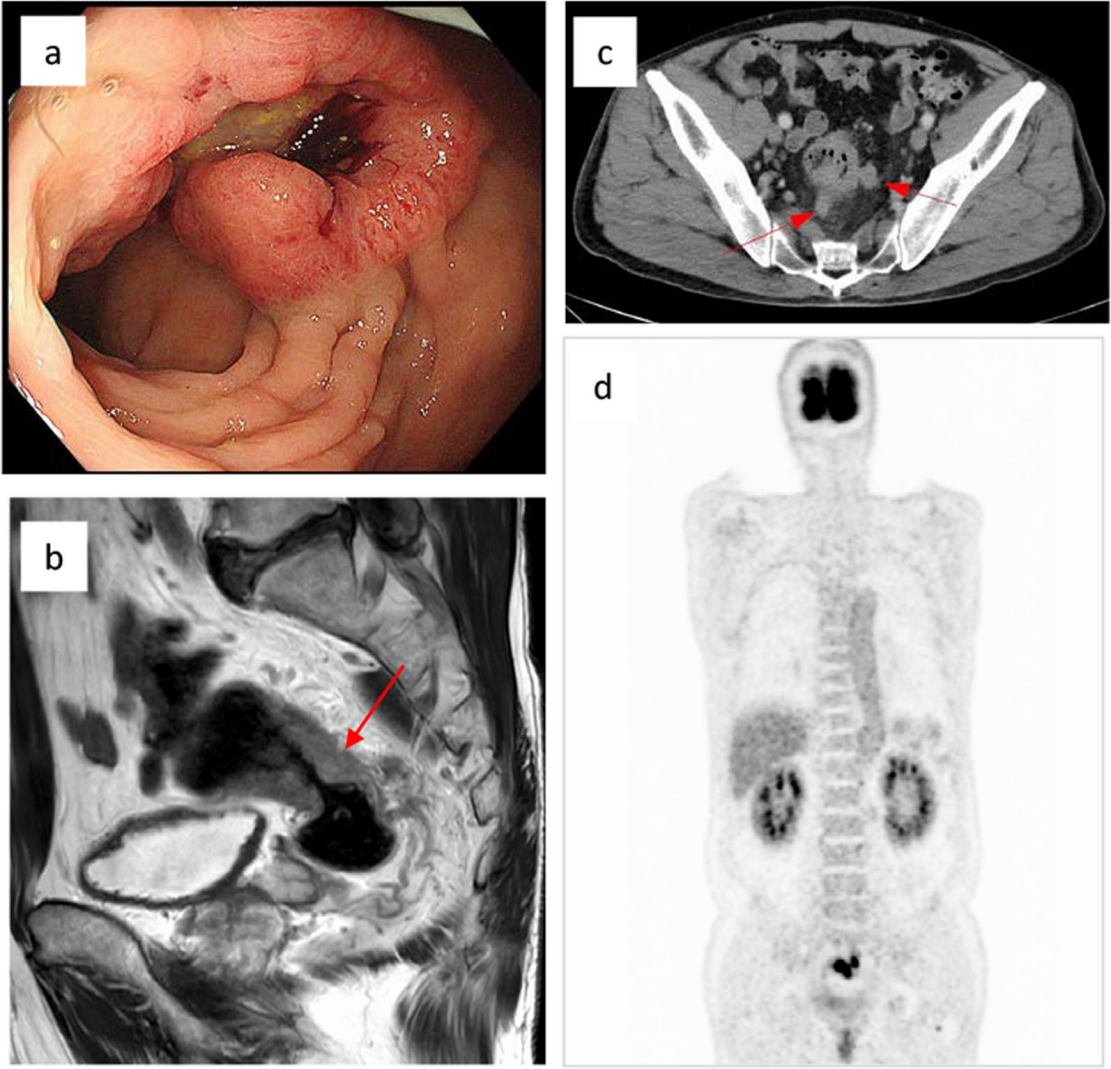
Preoperative image of rectal cancer. a Subcircumferential type 2 advanced carcinoma in the lower rectum. b Mid-sagittal plane of magnetic resonance imaging analysis of rectal cancer. c Abdominal computed tomography (CT) scan of circumferential wall thickening of the rectum and advanced metastasis to regional lymph nodes. d Positron emission tomography–CT showed no other obvious distant metastasis
